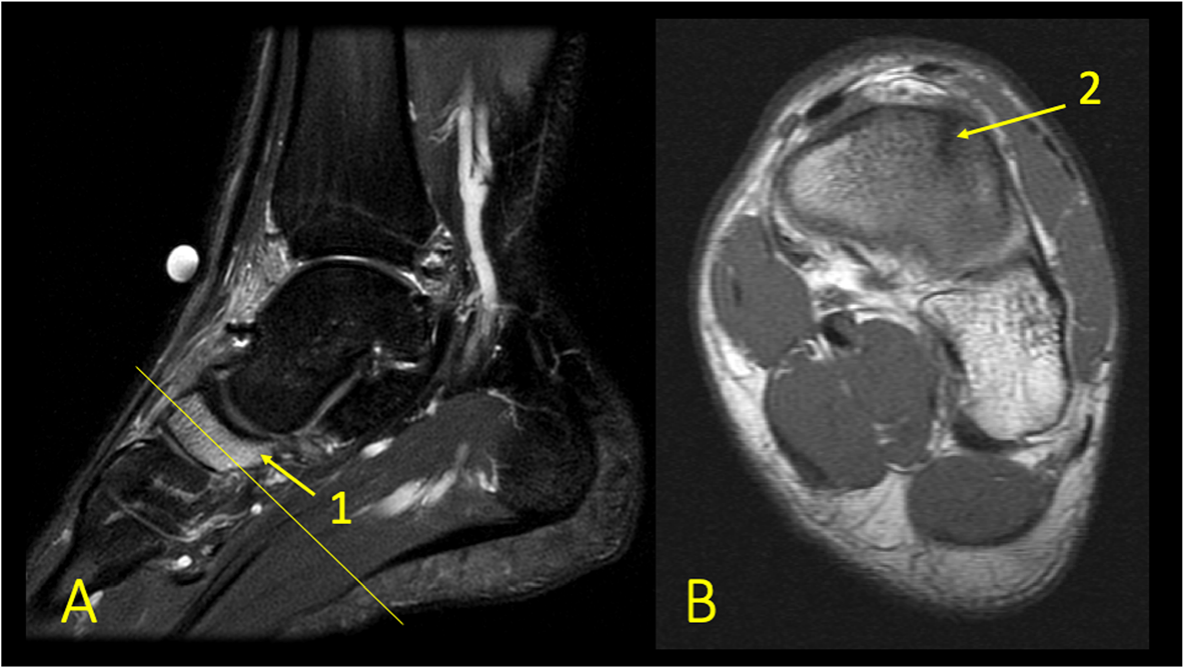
An 18-year-old female with diffuse pain at the level of the navicular bone. MRI with (a) T2-weighted fat suppression and (b) T1-weighted coronal images revealed extensive BME (1) and a stress fracture in the navicular bone (2)
